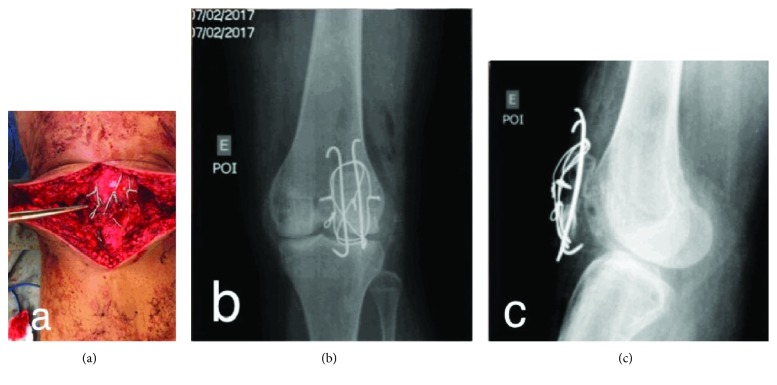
(a) Good contact between the fragments of the patella with autologous spongy graft. Radiographs in the anteroposterior (b) and lateral (c) views of the left knee in the immediate postoperative period.
